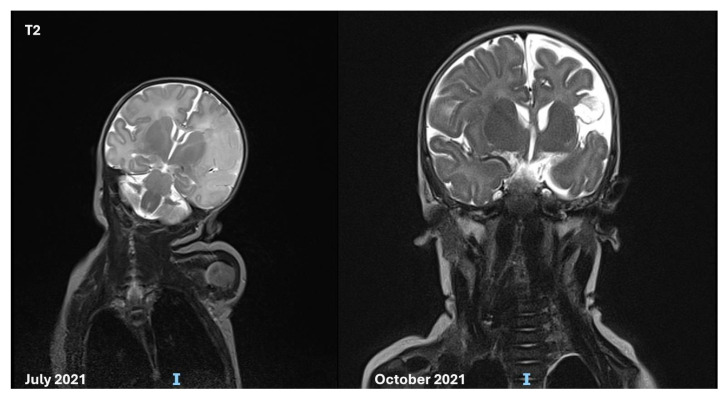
Brain MRI in T2 performed at birth, and after 4 months, showing recent and the subsequent lesions from an ischemic stroke in the deep and superficial cortical layers. Acute swelling and a marked reduction in the Sylvian cisterns were observed.
